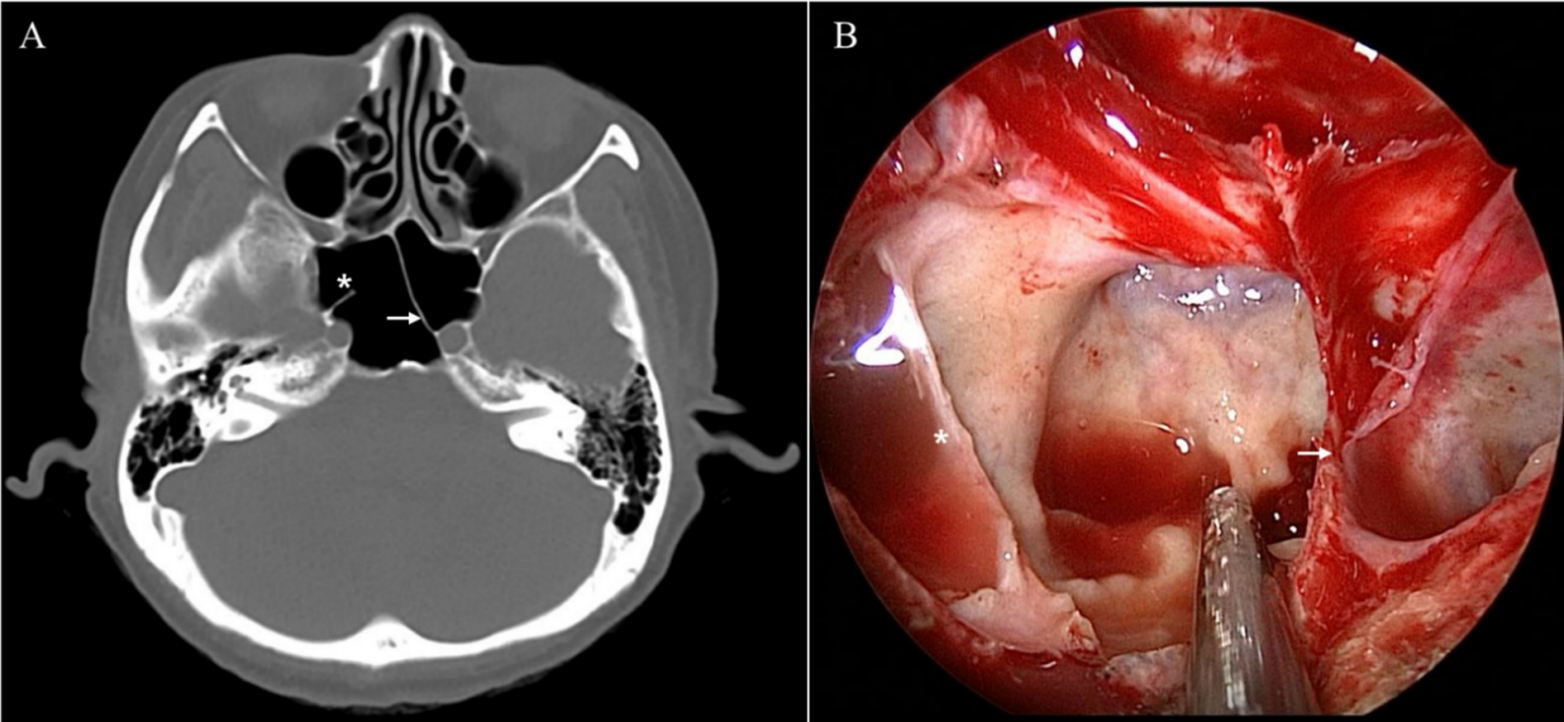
An intersphenoid sinus septum covering the paraclival internal carotid artery (asterisk) and entering that artery (arrow) in a patient with postsellar pneumatization, as revealed by preoperative paranasal sinus computed tomography (A) and during endoscopic endonasal transsphenoidal surgery (B).
